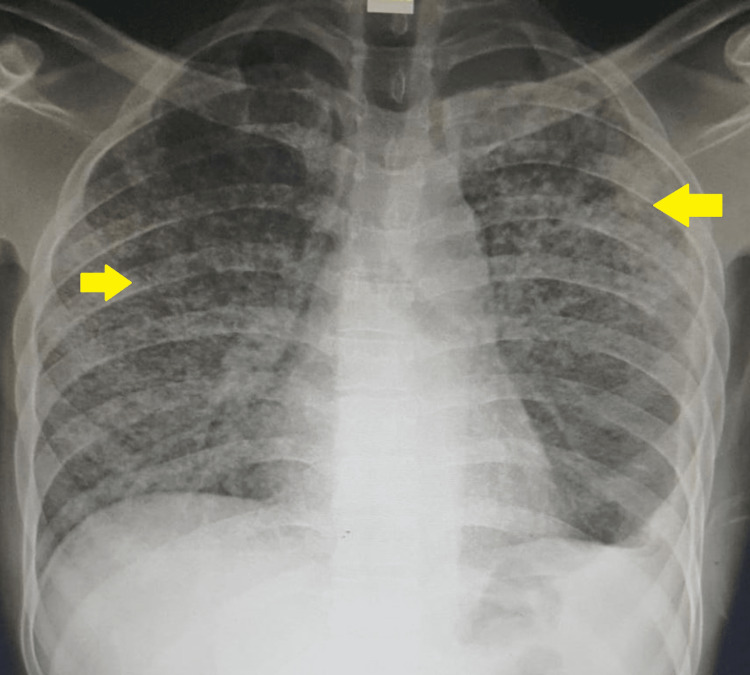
Chest X-ray with a posteroanterior (PA) view on admission showing bilateral symmetrical miliary opacities (yellow arrows).
